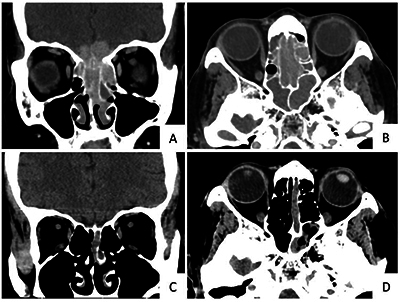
Typical location of olfactory neuroblastoma (ONB), shown in a 70-year-old woman presenting with anosmia. Contrast-enhanced computed tomography (CT) images showed ONB at the superior part of both nasal cavities with bilateral ethmoid sinuses and intracranial extension (A-B). Brain CT performed 4 years prior due to headache (C-D) showed soft tissue in the slightly expanded left olfactory recess, most likely representing a small ONB that went unrecognized at that time.
